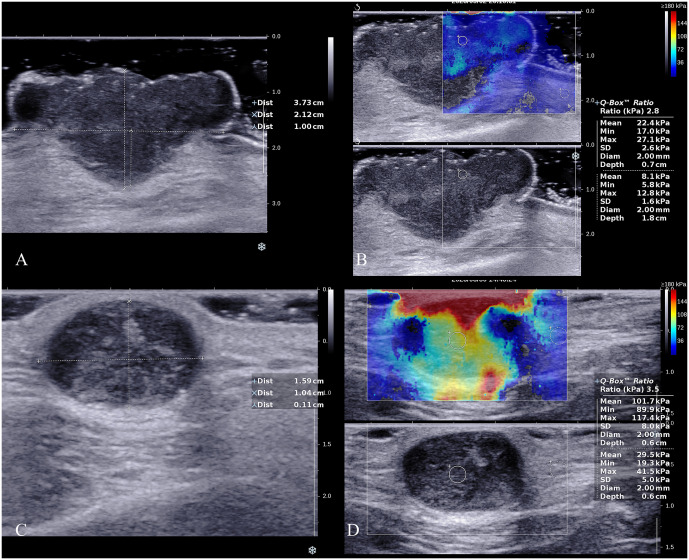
Representative high-frequency ultrasound (HFUS) and color Doppler imaging features of malignant (Squamous cell carcinoma) and benign skin tumors (Epidermoid cyst). (A) HFUS image demonstrating a hypoechoic tumor with multilayer involvement (epidermis, dermis, and subcutaneous tissue). The tumor exhibits irregular margins and heterogeneous internal echogenicity. (B) Color Doppler flow imaging of squamous cell carcinoma showing abundant intralesional vascularity with high-velocity blood flow signals. (C) HFUS image showing a well-circumscribed, anechoic to hypoechoic round tumor confined predominantly to the dermal layer with smooth margins and homogeneous internal echogenicity. (D) Color Doppler imaging of epidermoid cyst demonstrating minimal to absent internal vascularity.
